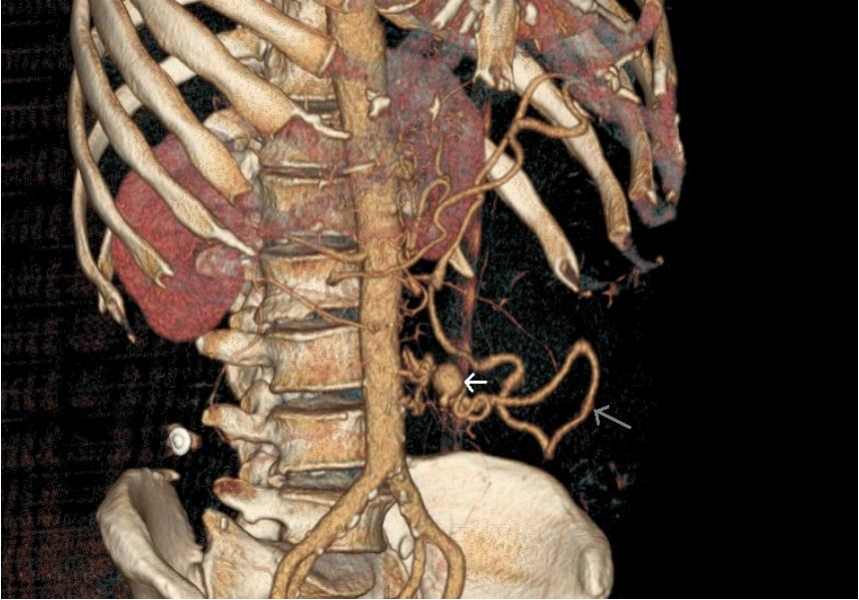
IMA aneurysm. CT reconstruction showing the inferior mesenteric artery aneurysm (white arrow), the artery tortuousness and a strongly developed marginal artery (grey arrow).
